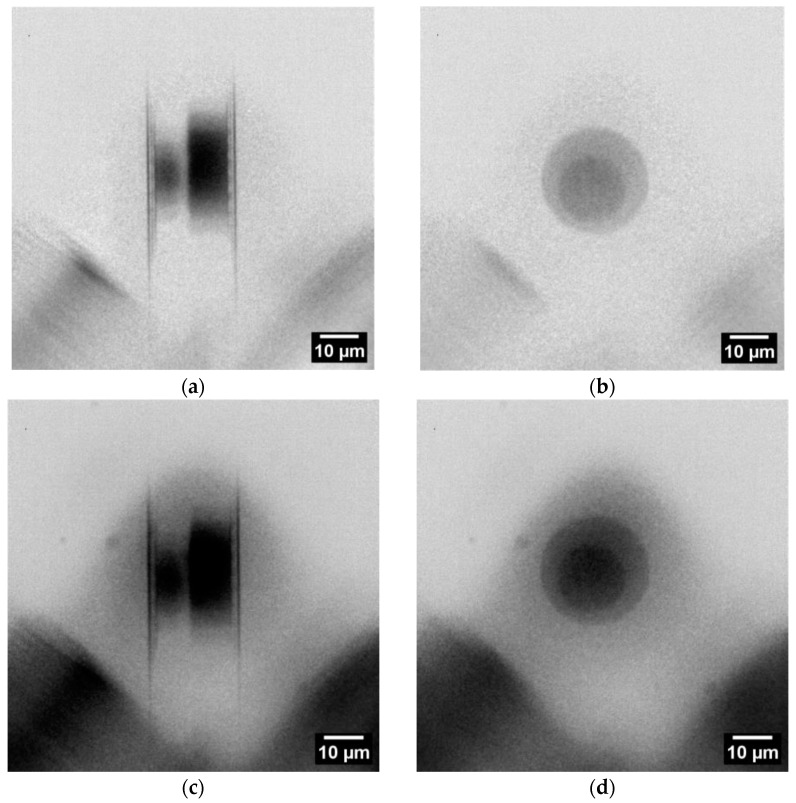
Radiographs of the SLID sample (rotated left by straight angle compared to Figure 3b) in two imaging directions: (a) at 90° cross-section view with visible solder of top and bottom dies and, in between, a light gray layer of IMC; (b) at 0° the plane view (stacking direction). (c,d) are similar to (a,b) with the addition of a 680 µm Si wafer piece in the beam path. Each radiograph is the result of five single radiographs with exposure time of 120 s using the camera binning 2 mode (1024 px × 1024 px).
